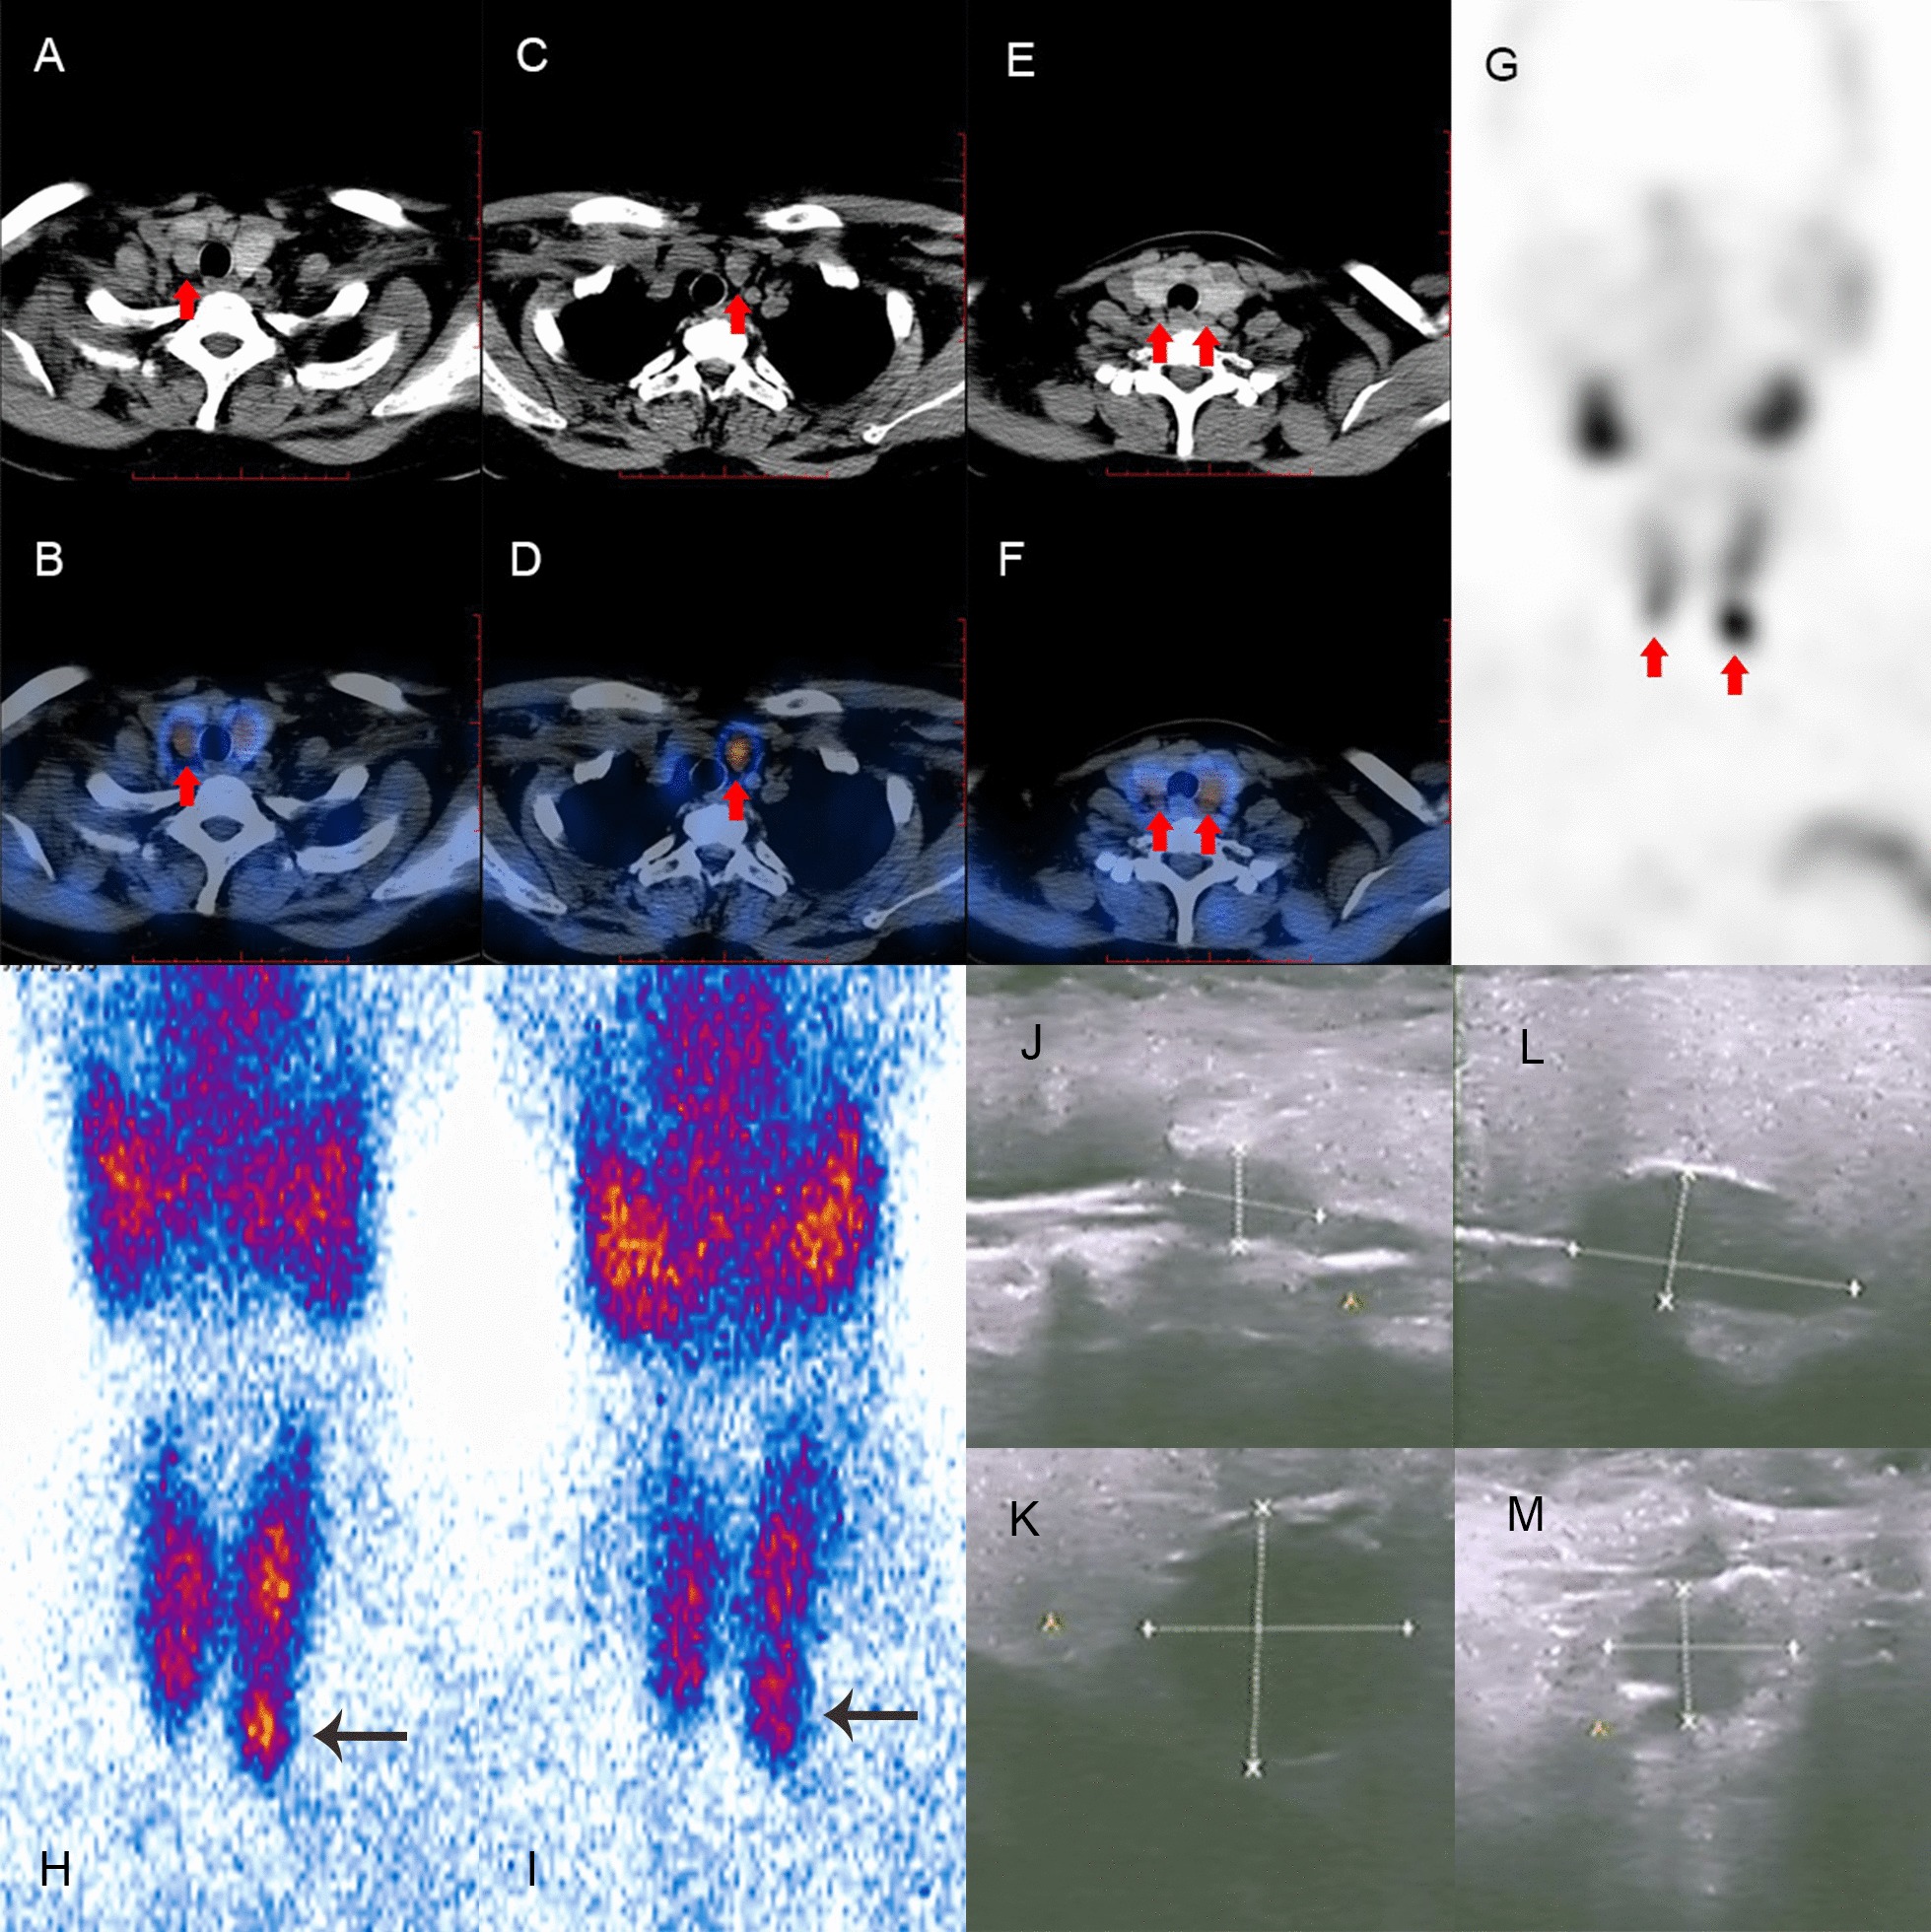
A middle-aged patient suffered from SHPT. The axial CT (a and c) and SPECT/CT (b and d) images showed two large soft-tissue masses with marked MIBI uptake in the back of the inferior poles of bilateral thyroid lobes (arrows). The axial CT (e) and SPECT/CT (f) images showed two small soft-tissue masses with slight MIBI uptake in the back of the upper poles of bilateral thyroid lobes (arrows). The maximum density projection image (g) only showed two foci in the inferior pole of bilateral thyroid lobes (arrows). Four lesions in the back of the thyroid gland were pathologically confirmed to be parathyroid hyperplasia. In the initial diagnosis, the lower left and right lesions were diagnosed correctly, but the upper left and right lesions were missed. The early and late planar images (h and i) showed MIBI uptake in the inferior pole of left thyroid lobe (arrows). US images (j–m) showed 4 enlarged parathyroid glands in the back of the upper and inferior poles of bilateral thyroid lobes.
